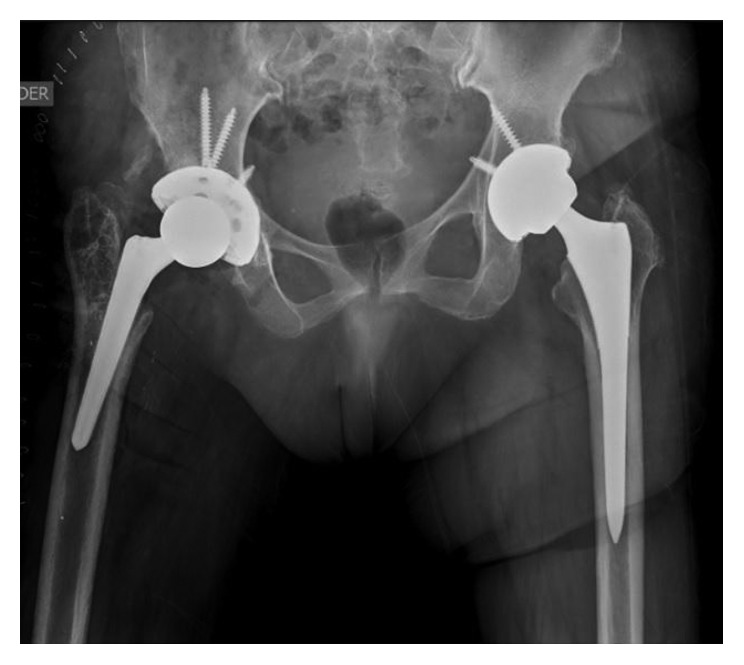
Postoperative radiograph after second stage and an uncemented total hip arthroplasty was performed.
